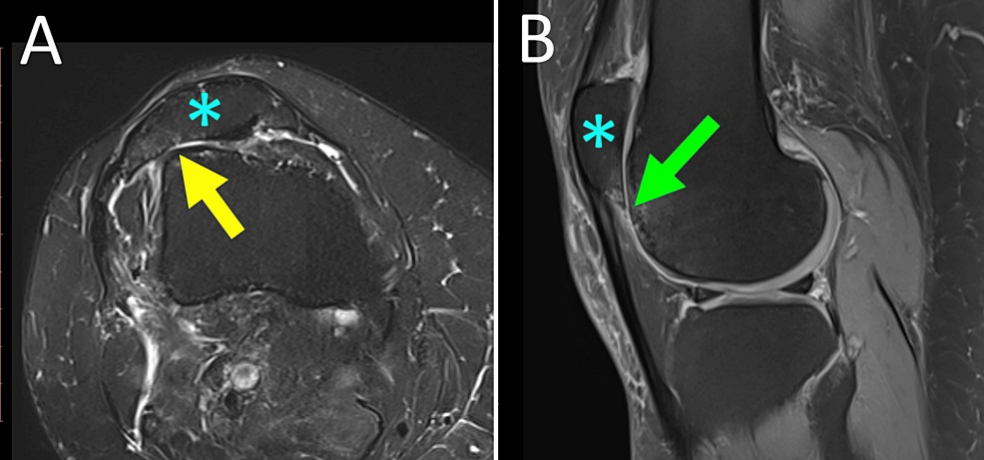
PFJ arthritis in a 48-year-old woman with chronic patellar instability and patella alta (A) Axial CT scan of the right knee. Lateral patellar subluxation (asterisk) with marked PFJ arthritis (yellow arrow) is evident. (B) Sagittal CT scan of the right knee. Patella alta (asterisk) and PFJ arthritis are evident (green arrow). Figure created by Panagiotis Samelis.
